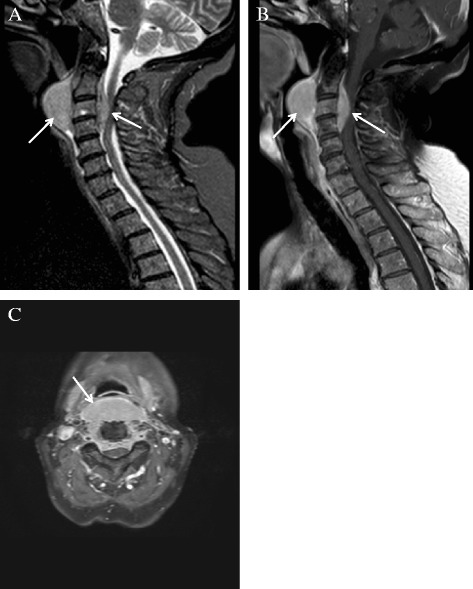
Cervical backbone magnetic resonance images showing a solid tumor of soft tissue extending from the spinal cord (arrows). A and B represent sagittal images, C represents the axial image.
